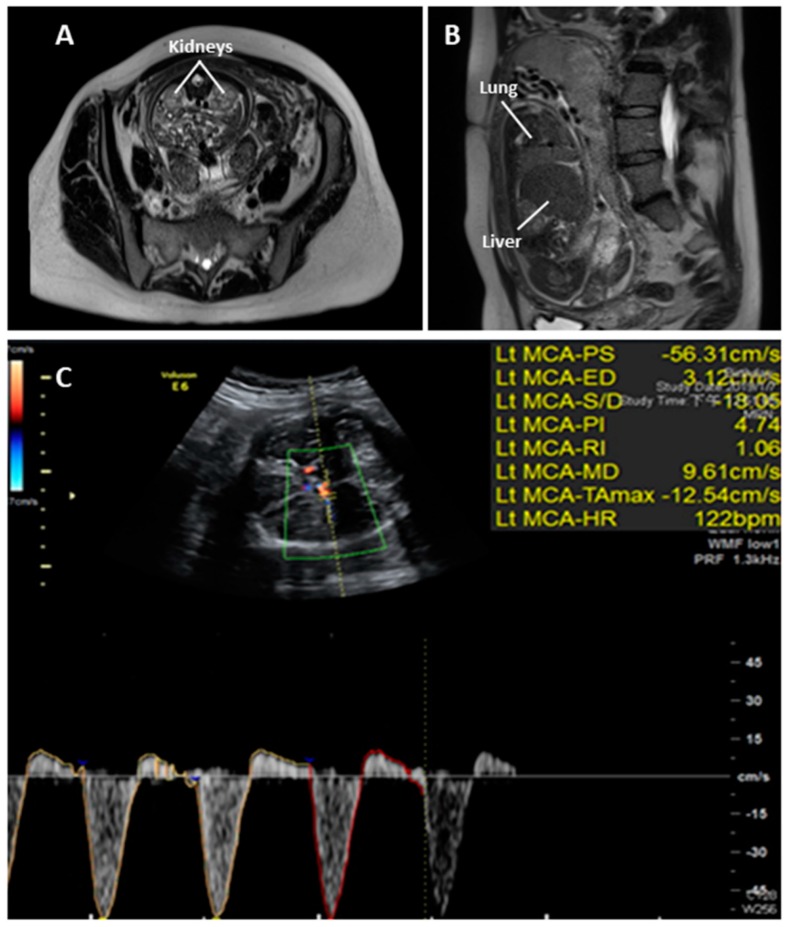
Magnetic resonance imaging (MRI) T2-weighted sagittal view of the fetus with RTD at 30^+5^ wGA showing (A) bilateral anatomically normal kidneys and (B) decreased signal intensity of the lungs, suggesting poor fetal lung maturation. Prenatal ultrasonography at 33^+2^ wGA showing (C) reversed end diastolic flow in the middle cerebral artery (MCA-REDF).
